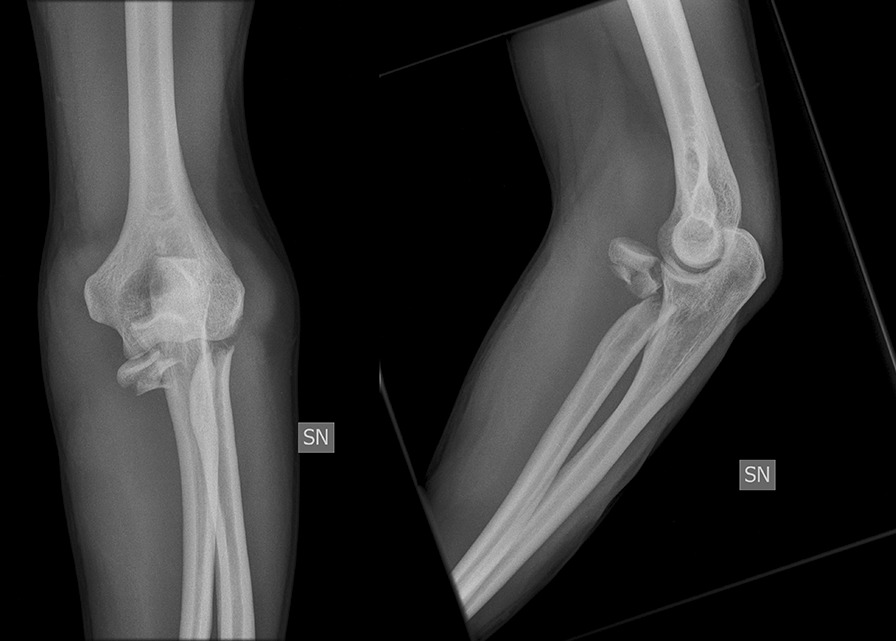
Radiographs of a comminuted radial head fracture
